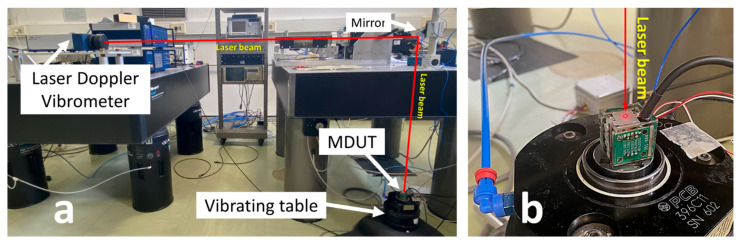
Characterization of the mechanical behavior of the embedding resin case during MDUT calibration in dynamic conditions: (a) the whole measuring system and (b) the MDUT on the vibrating table hit by the single-point Laser-Doppler vibrometer beam on the upper surface.
